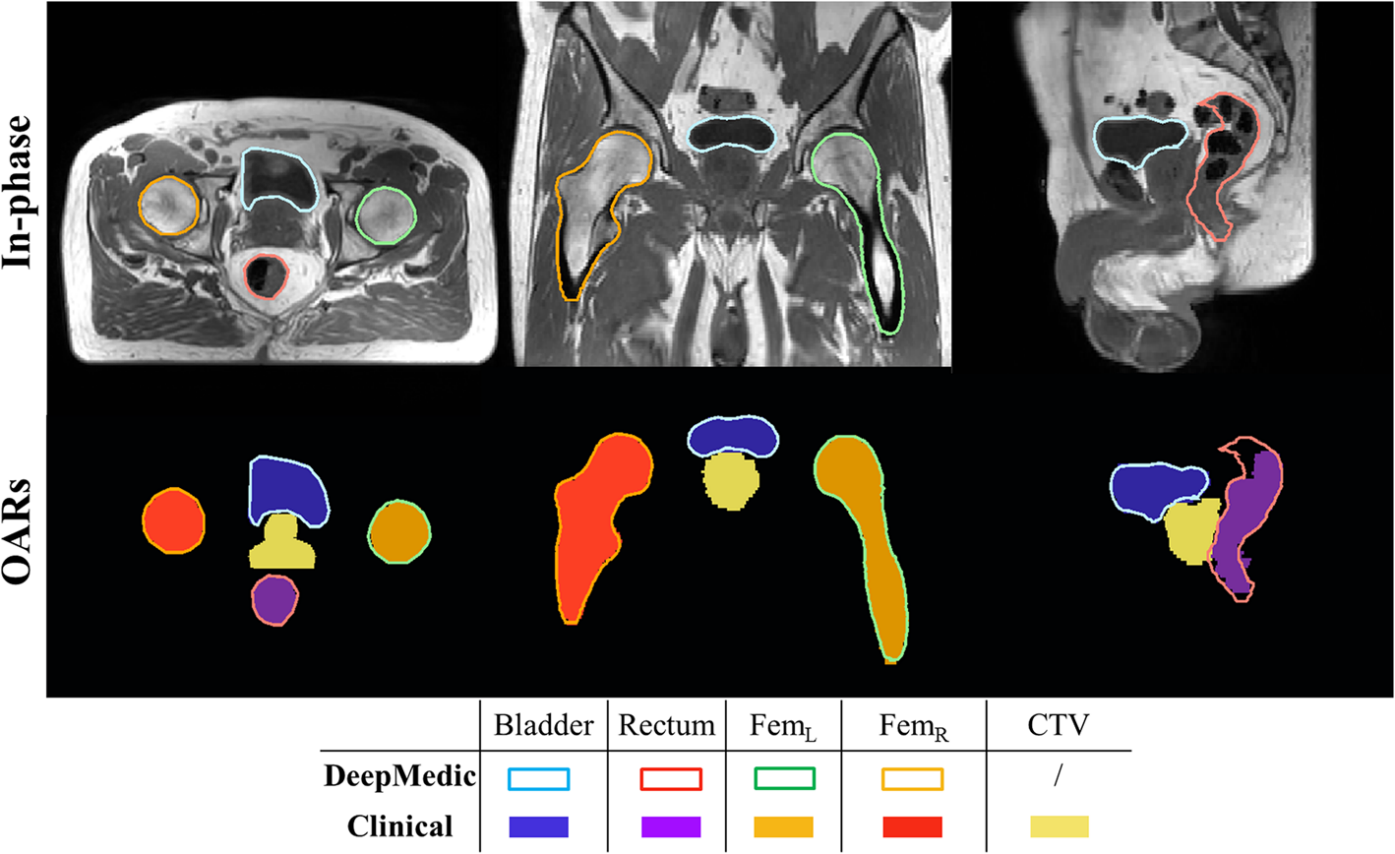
Example of in-phase MRI after cropping along with segmentations (OARs) obtained with DeepMedic (contours) versus clinical segmentations (filled contours) in the transverse (left), coronal (centre) and sagittal (right) view for a patient in the test. For this patient, average performance was obtained in terms of DSC: 0.96, 0.86, 0.97 and 0.97 for bladder, rectum, and femurs, respectively. Note that DeepMedic also outputs CTV, but it was not considered for clinical evaluation
